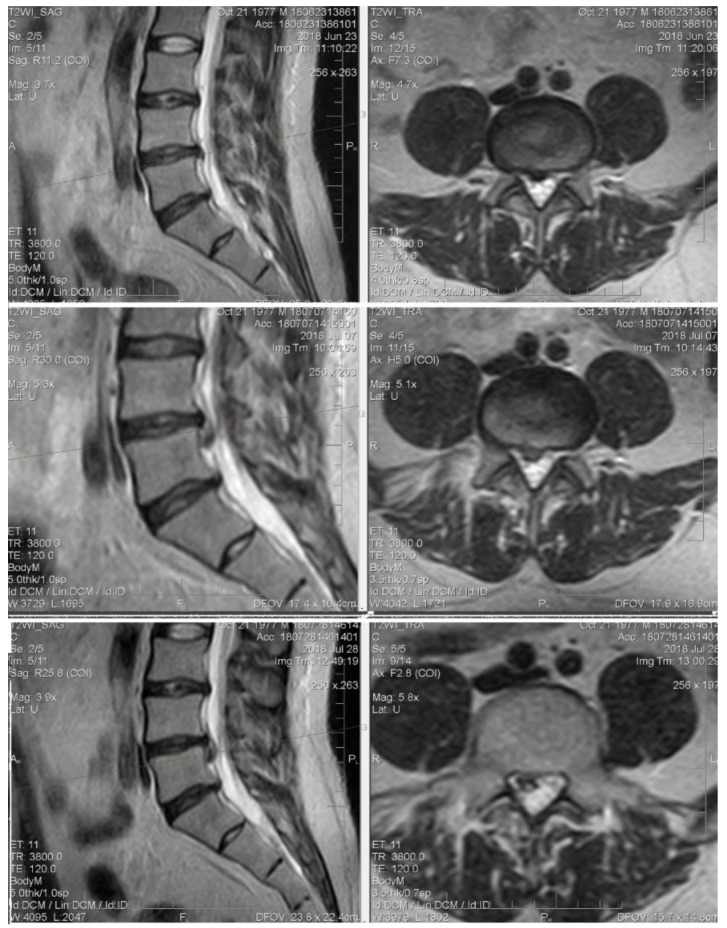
Initial T2-weighted MRI of the lumbar spine shows a large extruded disc at the L4-L5 level and the follow-up imaging shows up migrating fragment.
